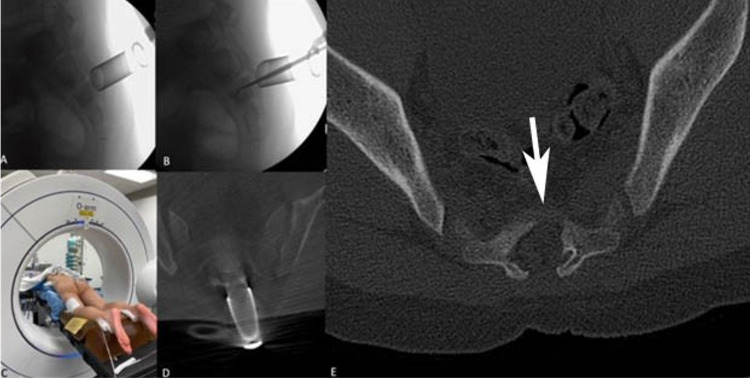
Repeat surgery showing positioning intraoperative lateral fluoroscopy to confirm final position of the retractor tube (A) and inspection of the extent of resection. Image showing O-arm placement (C) and confirming position (D); the postoperative CT scan shows extensive and complete resection (E)
